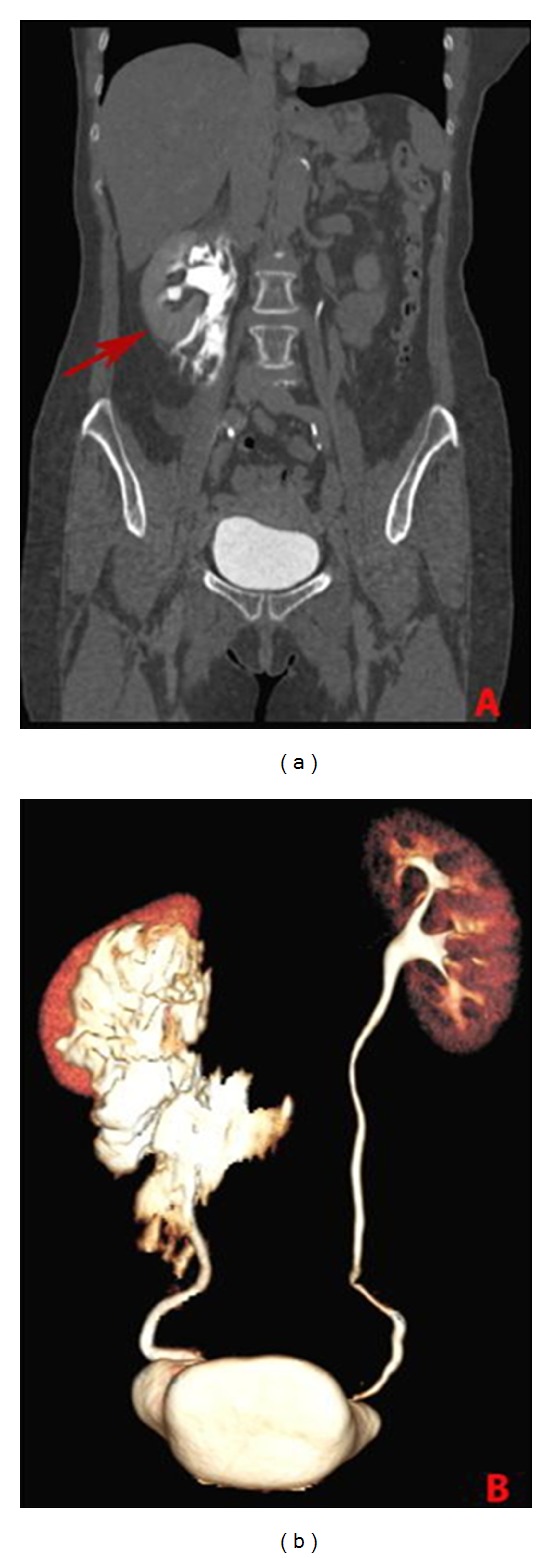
Contrast enhanced CT coronal image (a) and VR reconstruction (b) in the delayed phase documenting contrast extravasation extension (arrow).
